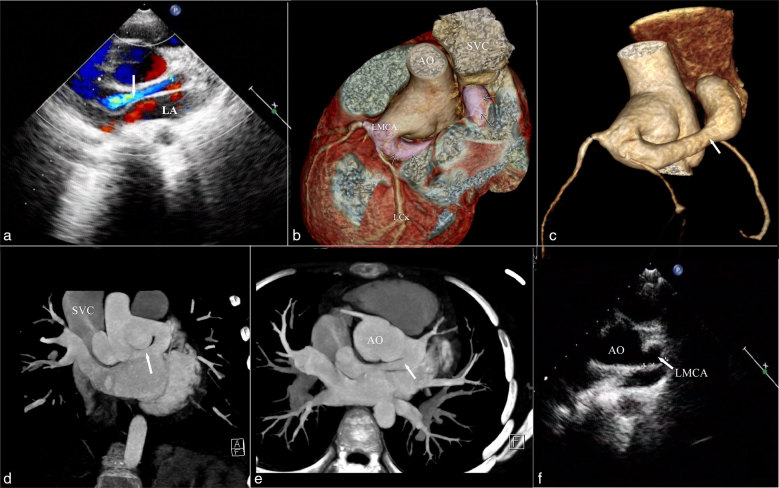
Pre-operative view (a–e). (a) Echocardiography showed a fistula in the parasternal long axis view. (b) DSCT with VR of the fistula, posterior view. (c) VR of coronary artery tree clearly demonstrating a connection between LMCA and SVC. (d) DSCT MIP, coronal view. (e) MIP, axial view. (f) Post-operative short-axis view of dilated LMCA (arrow indicates the closure of the fistula). Arrows in (a–e) indicate the fistula. AO, aorta; DSCT, dual-source CT; LA, left atrium; LMCA, left main coronary artery; MIP, maximum intensity projection; SVC, superior vena cava; VR, volume rendering.
